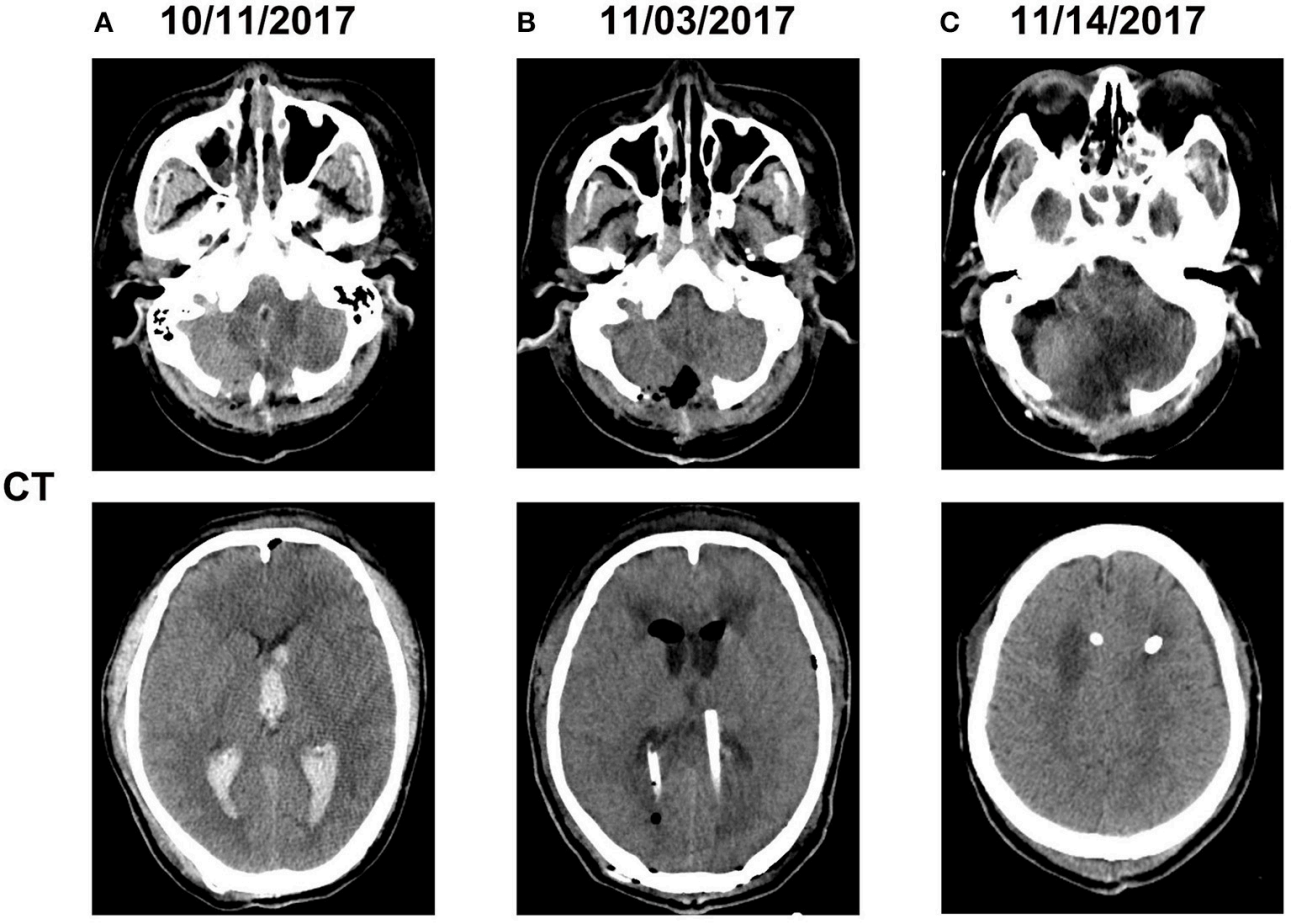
Computed tomography imaging before and after admission (A). The patient underwent hematoma removal from the cerebellum and decompression in a local medical facility. Computed tomography after admission to our hospital revealed cerebellar hemorrhage in the bilateral and 3rd ventricles (B). We temporarily placed a bilateral ventricular drainage in the occipital horns after the clearance of abscess on a ventriculoscope on November 3, 2017 (C). We replaced the bilateral ventricular drainage in the frontal horns due to CSF leakage in the occipital horns on November 14, 2017.
